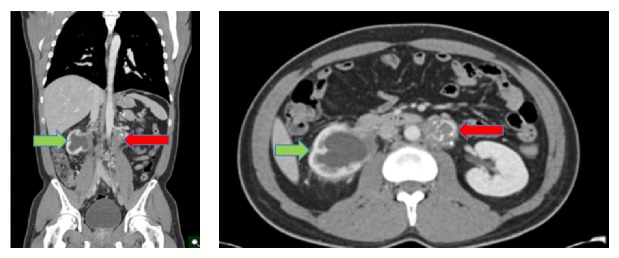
CT scan obtained 8 months after presentation status postchemotherapy showing reduction in the soft tissue stranding and thickening of the right kidney (green arrows) as well as stability of the retroperitoneal lymph nodes (red arrows).
